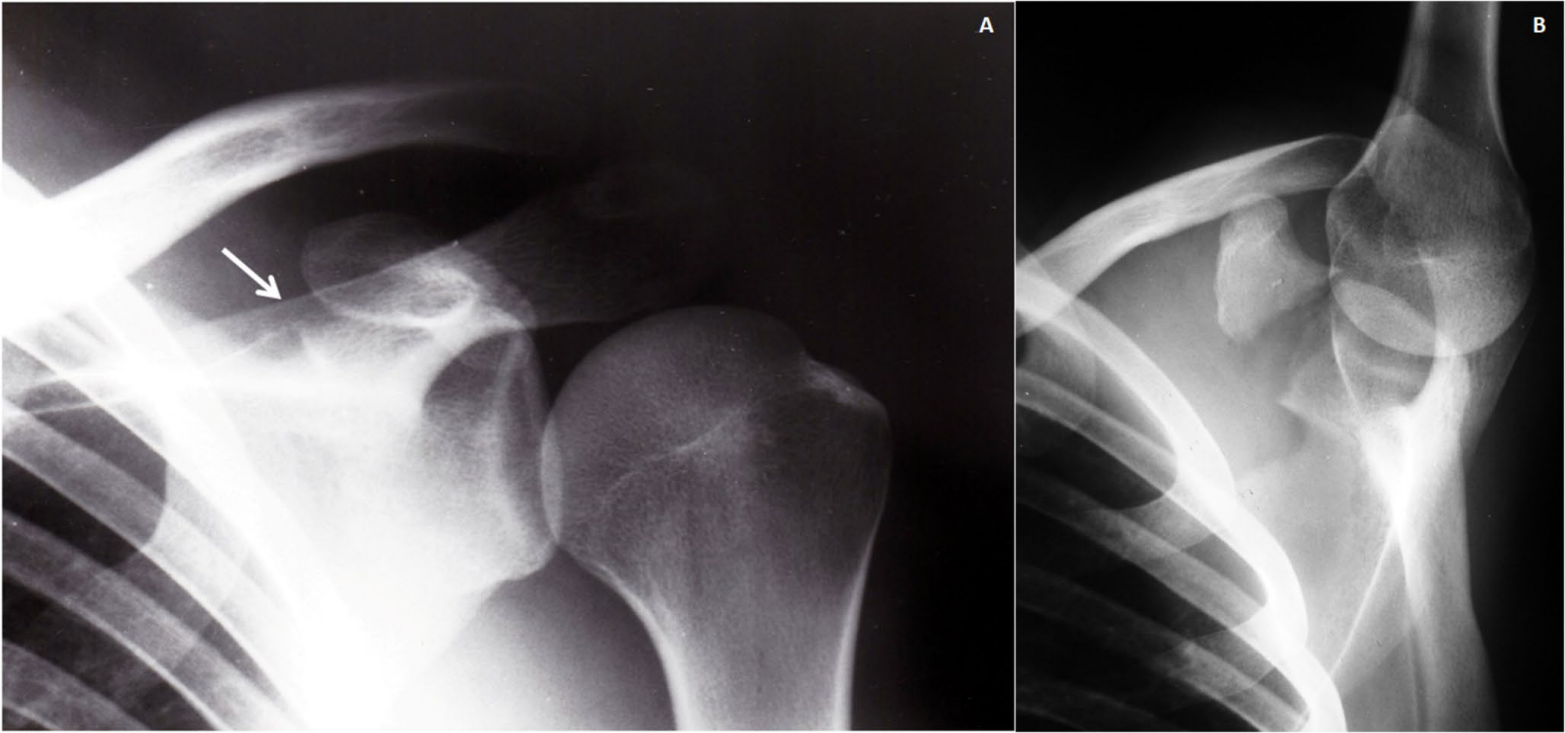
Case 3 Table 1. 4A: Antero-posterior radiograph of the left shoulder of a 32 years-old male shows an acromioclavicular dislocation with and associated CP base fracture (arrow). 4B: An abduction view shows more clearly the displaced CP base fracture without overlapping with other bony structures. Coracoclavicular distance is normal confirming CC ligaments were intact
